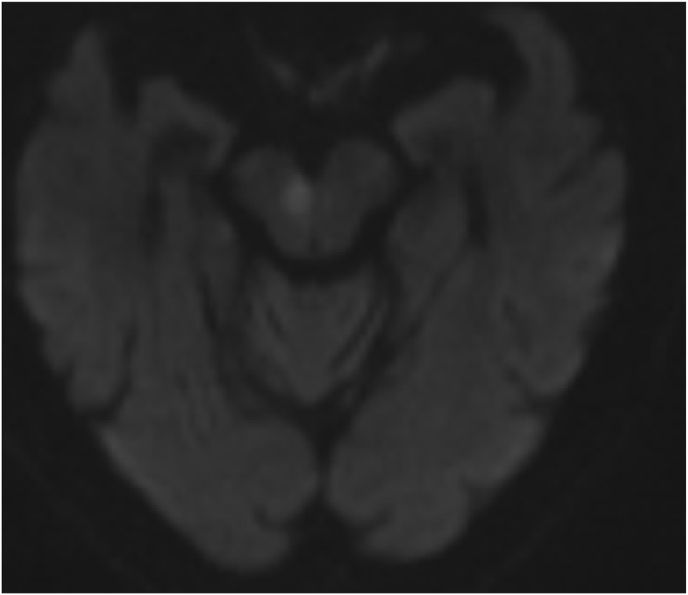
DWI showing hyperintense right midbrain infarction.
